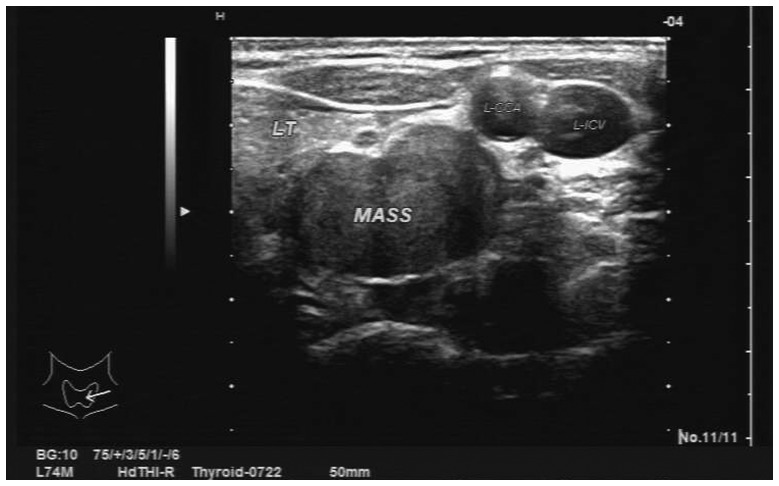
Ultrasonography revealed multiple bilateral thyroid nodules and a hypoechoic mass in the left side of the neck.
